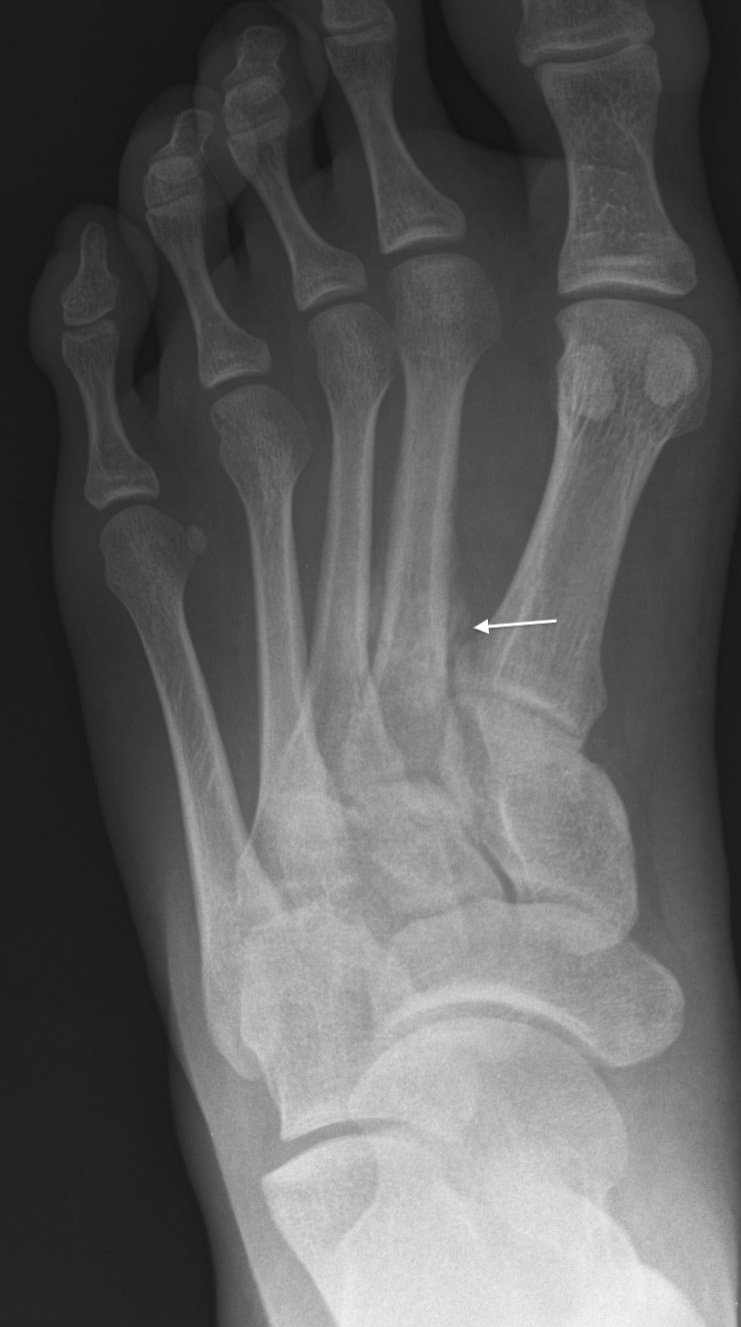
Plain film radiograph of 20-year-old patient with foot pain associated with exercise. Subtle periostial reaction indicated by arrow at base of second metatarsal bone indicates stress fracture.
